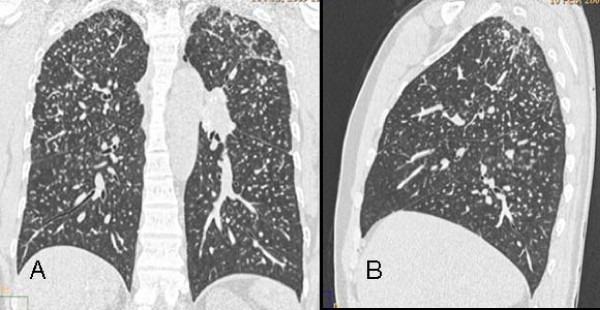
a, b - Coronal (a) and sagittal (b) reconstructed computed tomography images showing the predominance of nodules located in the upper lobes and peripheral regions of the lungs in a 29-year-old man who worked as a denim sandblaster for 20 months.
